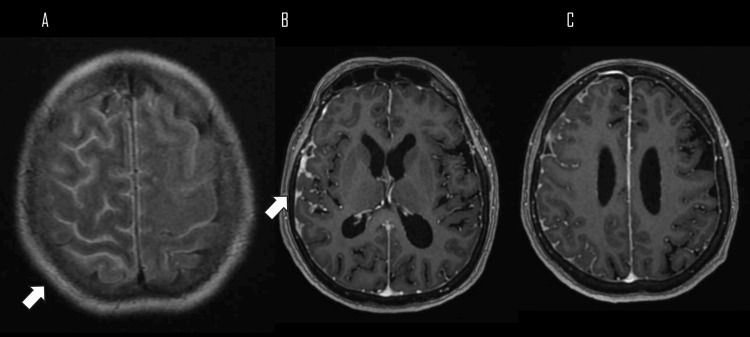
MRI of the brain in T1-weighted contrast-enhanced sequence in axial sections, showing involvement of the leptomeninges of the right cerebral hemisphere. (A) Axial section at the cortical level showing leptomeningeal hyperintensity (arrow) in the frontal lobe and part of the right parietal lobe. (B) Axial section at the level of the anterior horns showing gadolinium enhancement of the leptomeninges in the insular region (arrow). (C) A slide at the level of the ventricular bodies shows increased gadolinium enhancement in the leptomeninges at the frontal lobe, parietal lobe, and midline.
